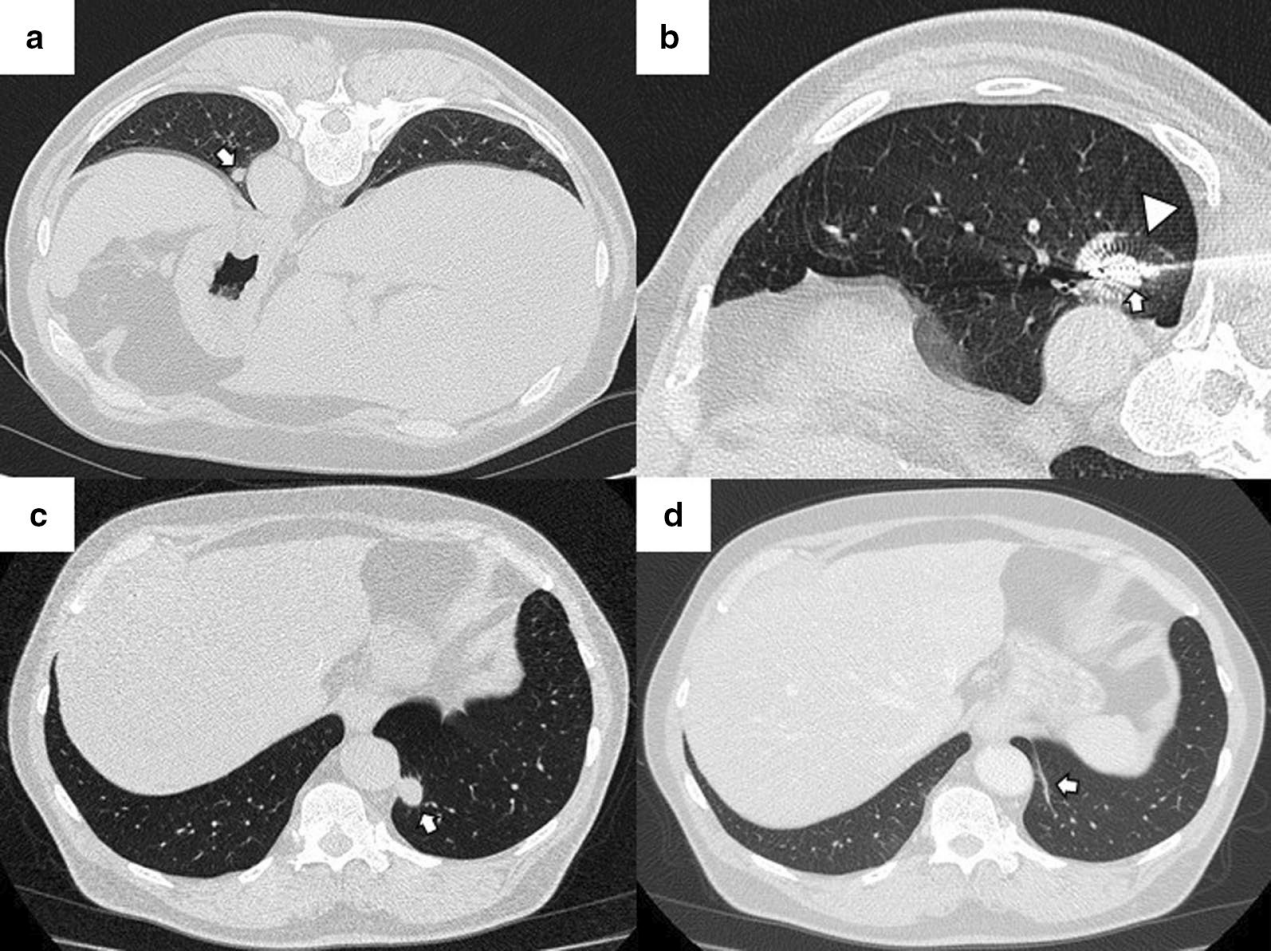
"Iceball" formation while performing an image-guided percutaneous lung CA. a CT of a patient with oligometastatic disease due to a small cell renal carcinoma with a lesion near the descending thoracic aorta (arrow). b CT-guided CA of this lesion, given the lesion's small size, it was possible to use a single straight probe placed in the center of the lesion (arrow). Note the hypodense halo surrounding the lesion, consistent with the “ice ball” formed during treatment (triangle). c Non-contrast CT scan one month after treatment. The treated area shows a larger size than the original lesion (arrow). However, it is a rounded area of similar size to the image immediately after treatment (not available). d Non-contrast CT scan six months after treatment. A lung scar is now observed in the treated area, consistent with effective treatment (arrow)
